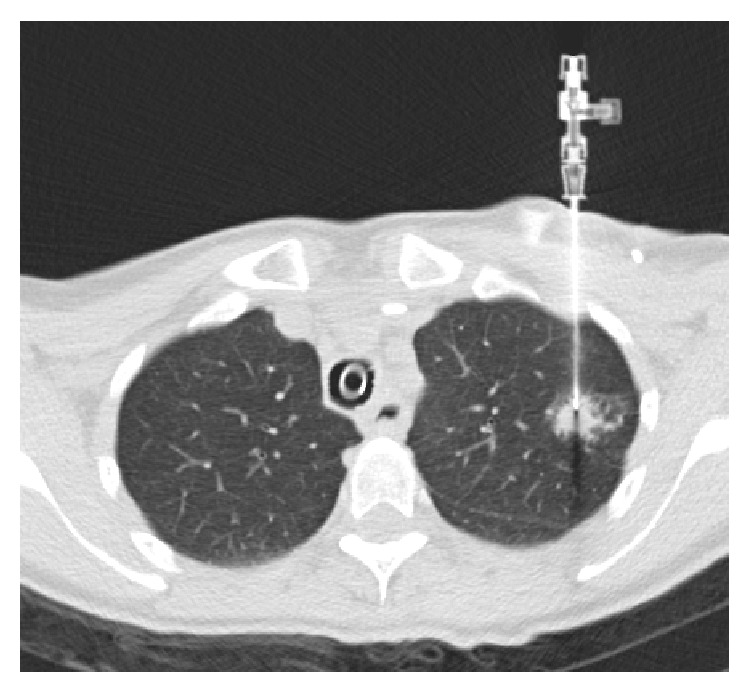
CT image during lung biopsy with guiding needle combined with hemostatic valve as described (under intubation anaesthesia at the explicit request of the patient, with venous port system visible in the edge region).
